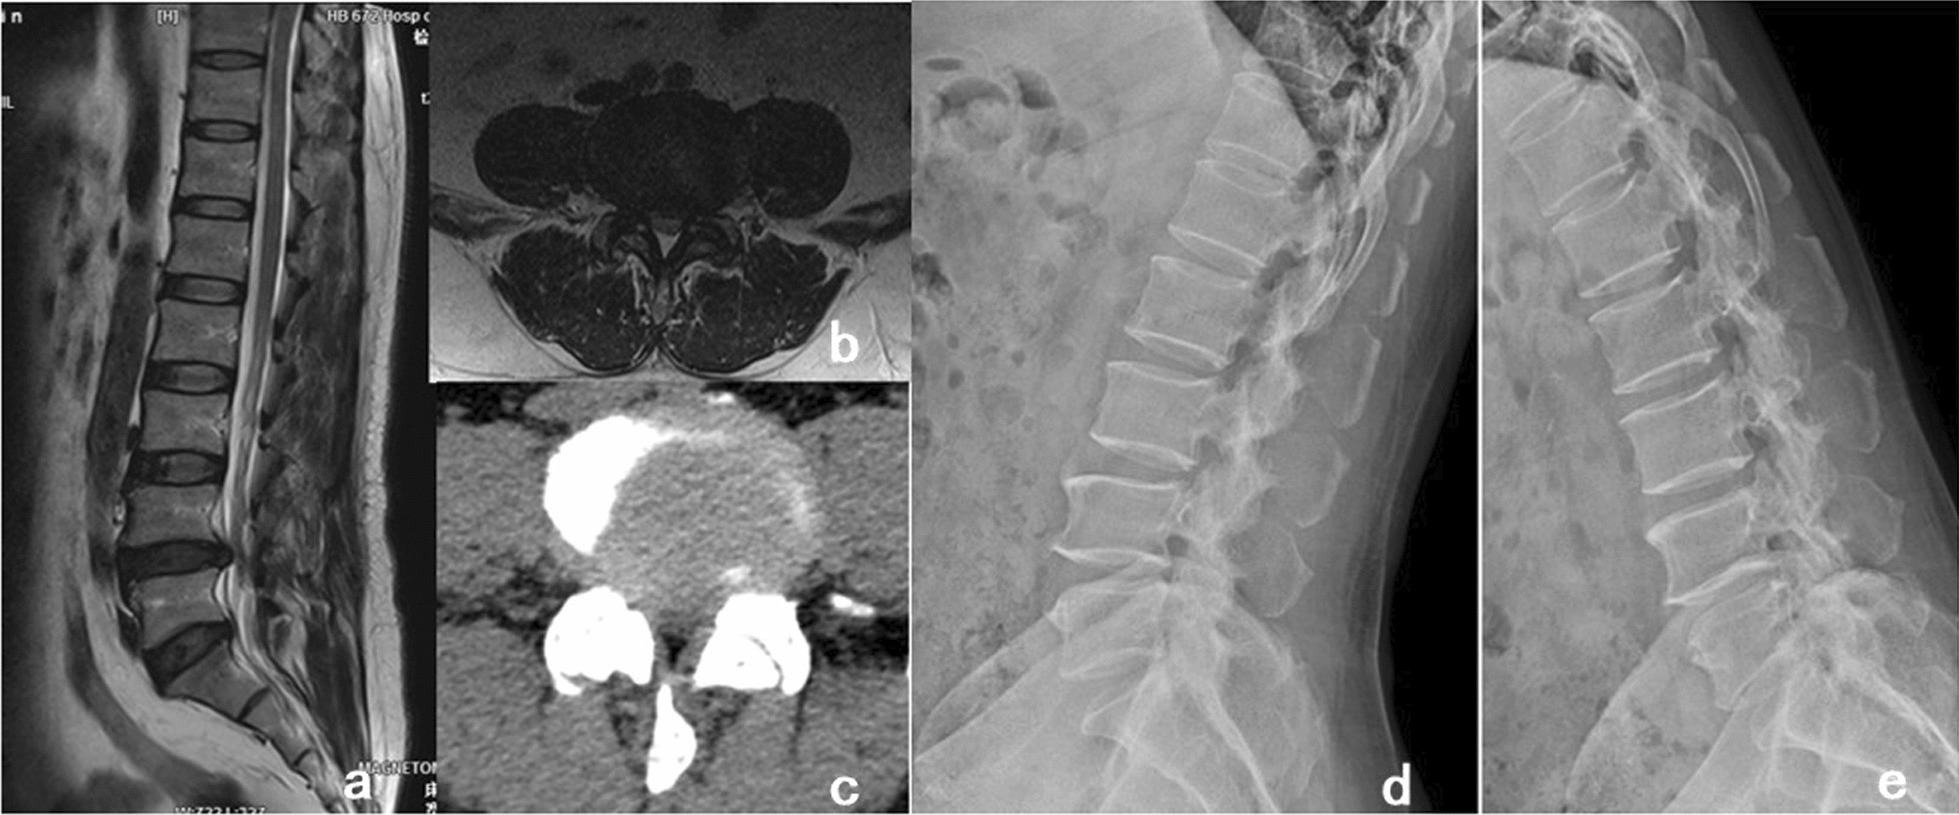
a, b Pre-operative MRI in sagittal and coronal positions showed a herniated disc at L4/5 with the hyperplastic ligamentum flavum; c Pre-operative CT in coronal position showed a herniated disc at L4/5 with the narrow spinal canal; d, e Pre-operative dynamic X-ray radiographs showed the stability of lumbar spine was good
